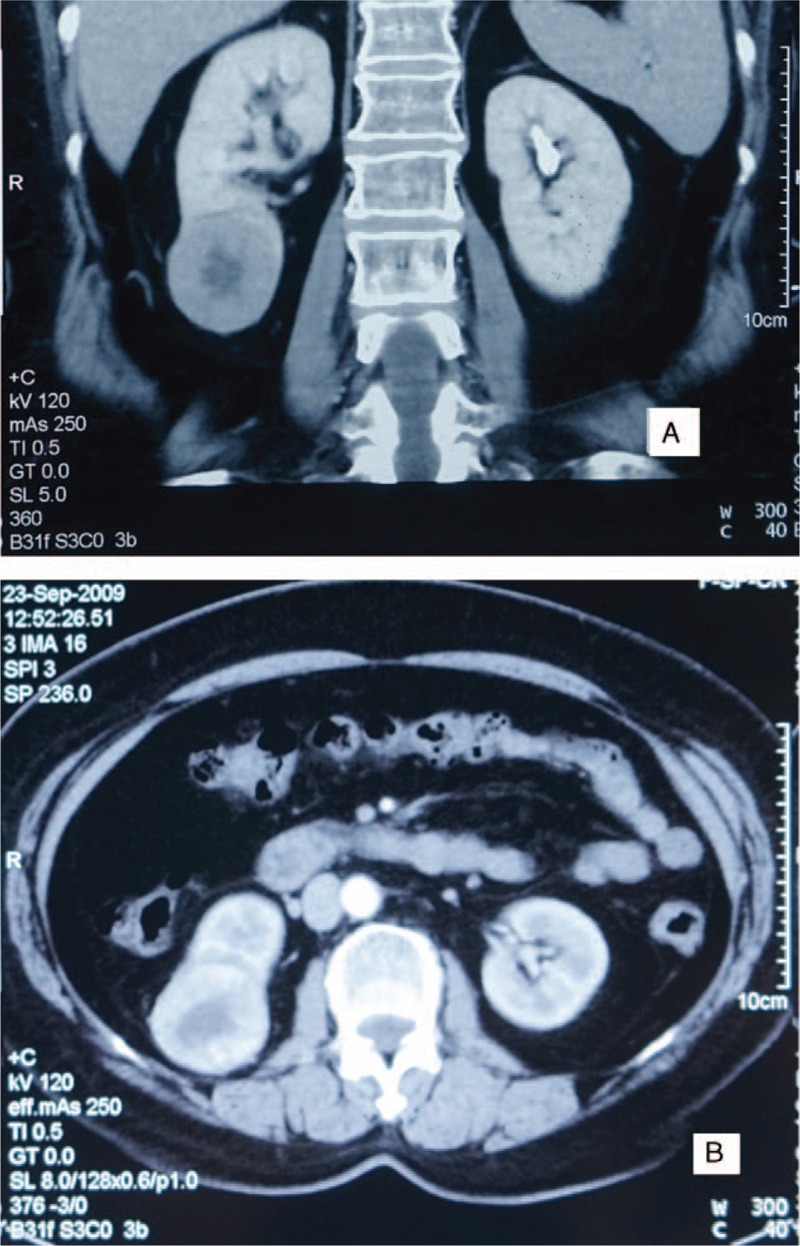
CT scans of TLFCK. (A) Coronal and (B) axial CT scans with contrast showing a 5 × 4.5 × 3-cm inhomogeneous and partially exophytic lesion in the right lower kidney, with hypodense, necrotic lesions in the middle surrounded by relatively hyperdense lesions. CT = computed tomography, TLFCK = thyroid-like follicular carcinoma of the kidney.
